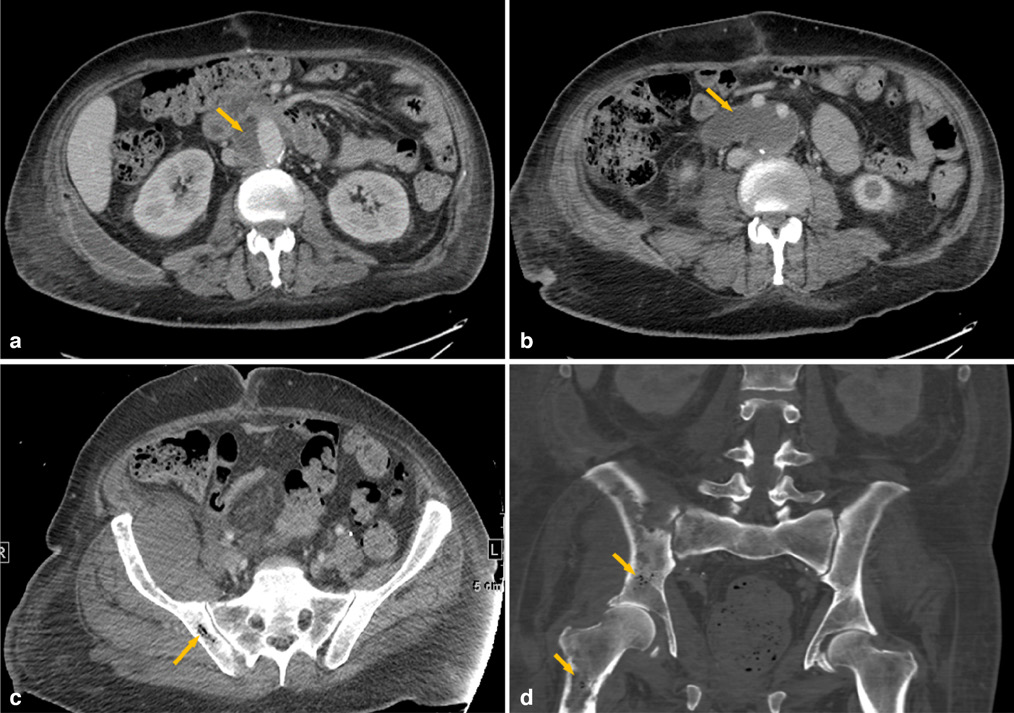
Post-contrast axial CT scan of the abdomen (a, b) showing periaortic fluid collections surrounding the infrarenal aorta down to the aortic bifurcation (arrows). Intraosseous pneumatosis with the ‘pumice stone’ sign typical of EO and bony lytic changes are seen in the right-sided pelvic bones and the proximal right femur (c, d). The right iliac crest muscle is swollen with surrounding fat stranding, corresponding with the findings seen on MRI in Figure 2. EO, emphysematous osteomyelitis.
